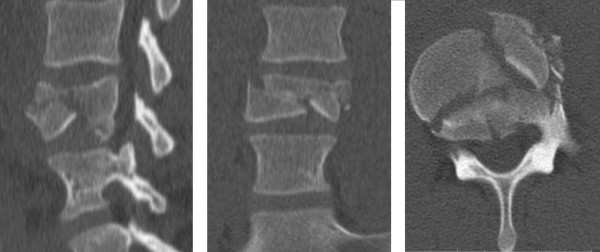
Preoperative computed tomography imaging. There is a lumbar L4 burst fracture with minimal loss of height and canal compromise.
